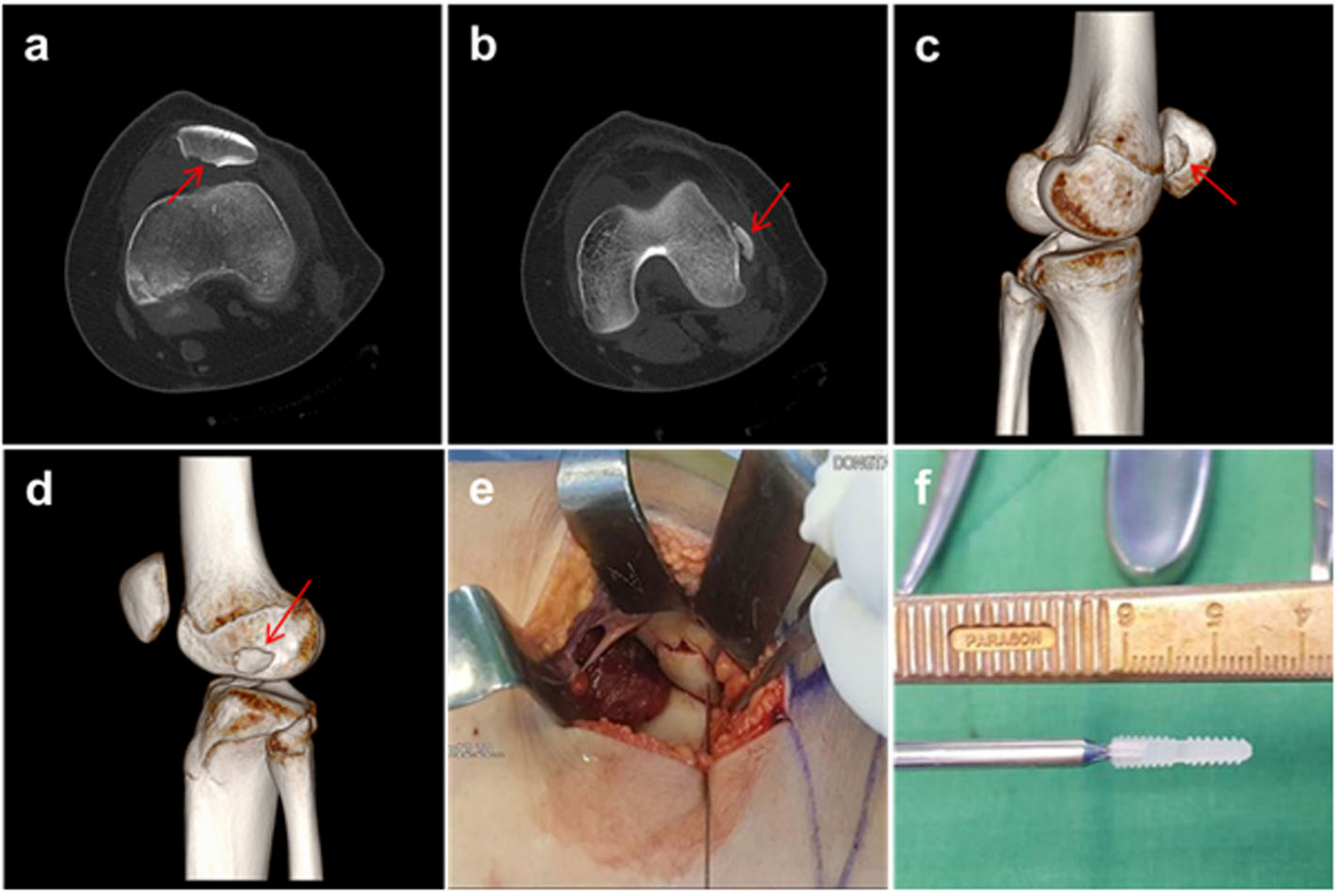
A 19-year-old female with OCF from the inferomedial facet involving central ridge. a A CT axial image showing osteochondral defect on the patellar inferomedial facet involving central ridge (arrow). b Osteochondral fragment next to LFC (arrow). c A 3D-CT reconstruction image showing osteochondral defect on the patella inferomedial facet involving central ridge (arrow). d Osteochondral fragment next to LFC (arrow). e, f Arthroscopic retrieving the fragment and open refixation of the fragment with a biodegradable headless screw
